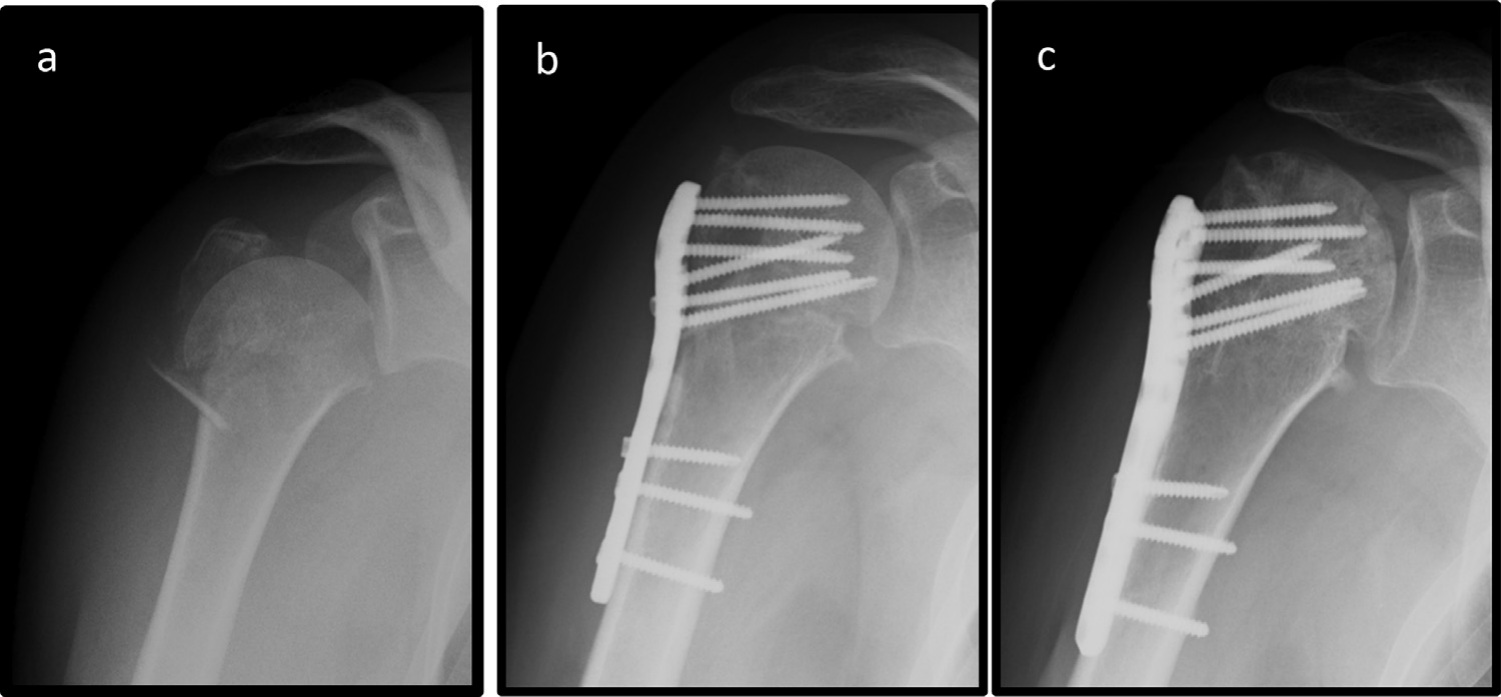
Radiographs showing initial fracture (a); one day after the surgery (b); six months after the surgery with radiological evidence of HHO (c).
